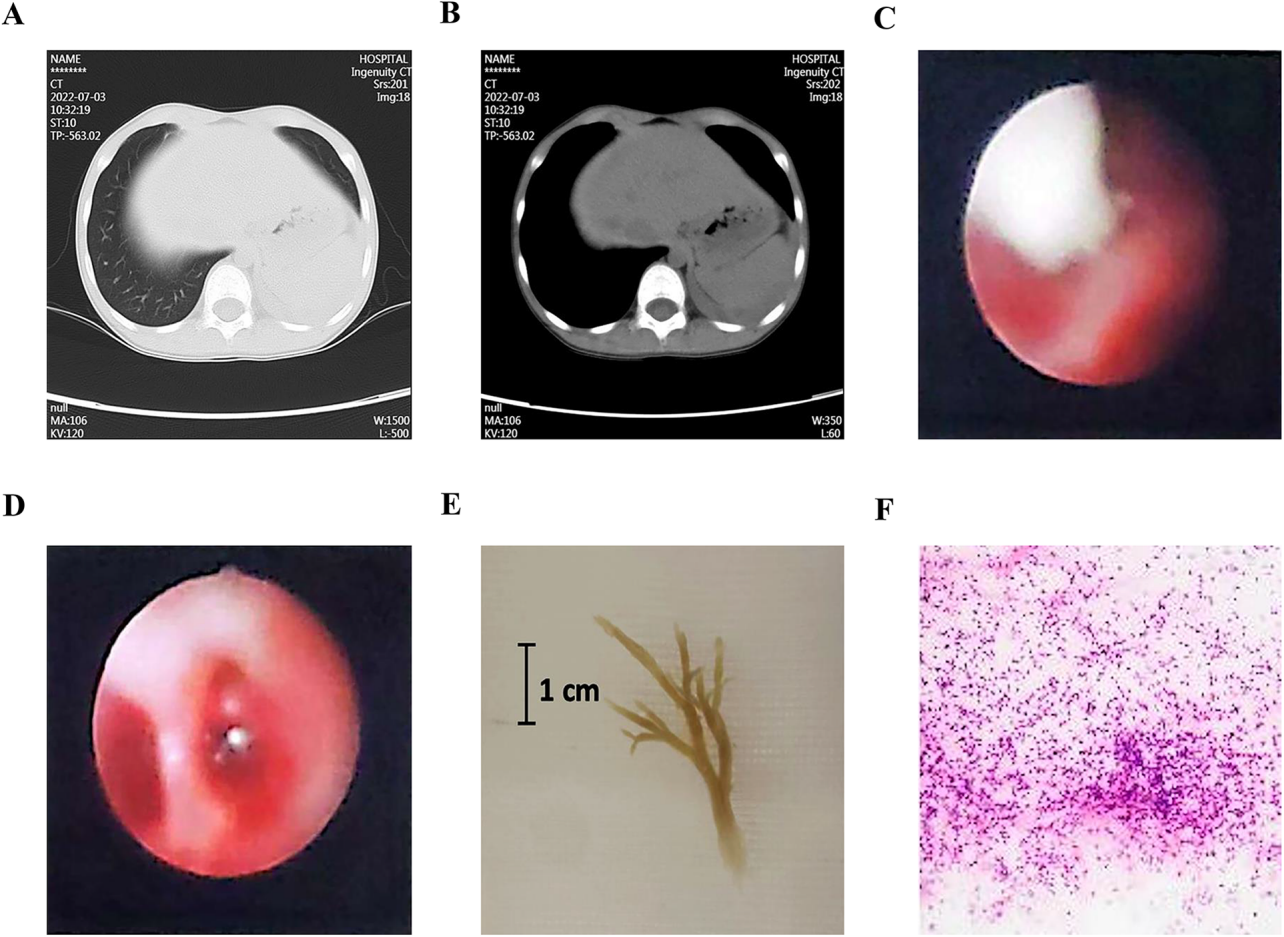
A representative case with plastic bronchitis and pleural effusion. (A,B) Chest CT images showed an obstruction of the proximal bronchi in the left lower lobe, atelectasis and consolidation, and pleural effusion. (C) Bronchoscopy noticed a white mucus plug. (D) After the removal of the plug, congestive and swelling mucosa were observed. (E) A cast of plastic bronchitis was removed. (F) Cytopathology of bronchoalveolar lavage fluid revealed 60% neutrophils, 30% phagocytes, and 10% ciliated columnar epithelial cells.
